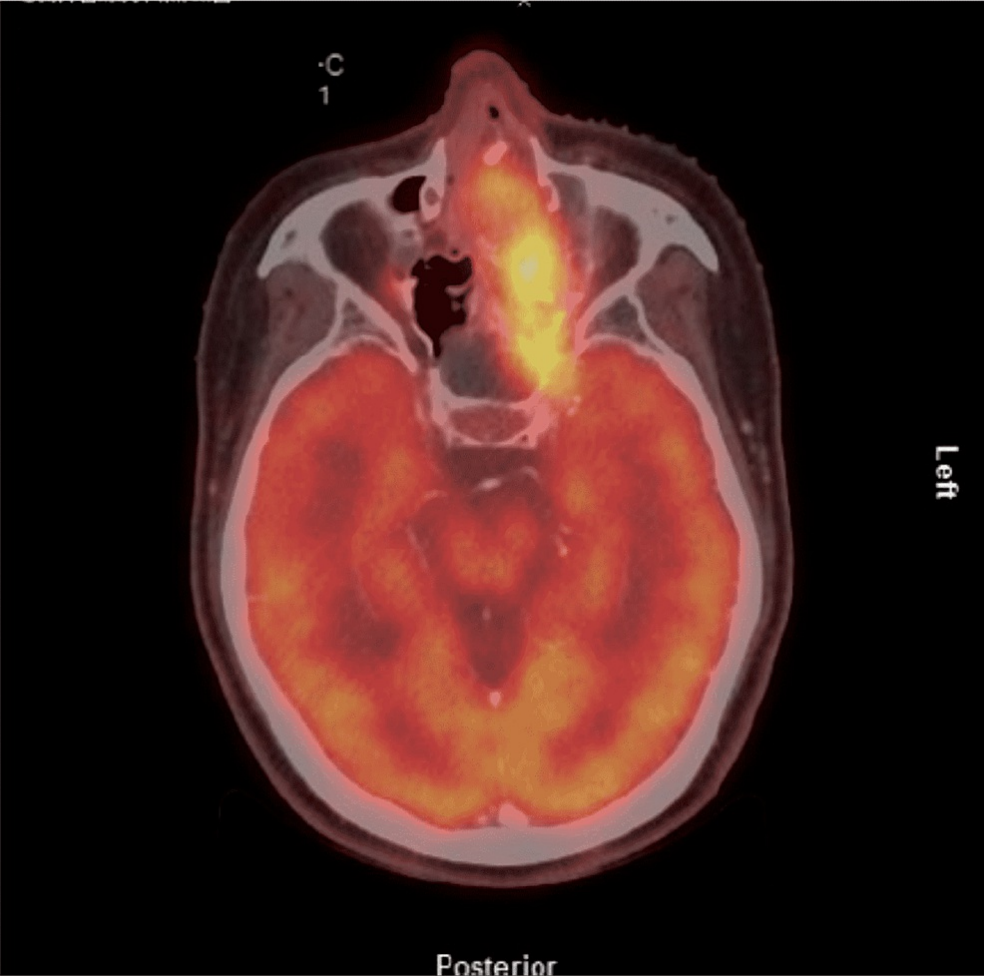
Positron emission tomography (PET) CT demonstrating a significant FDG uptake of metabolically active lesion.
